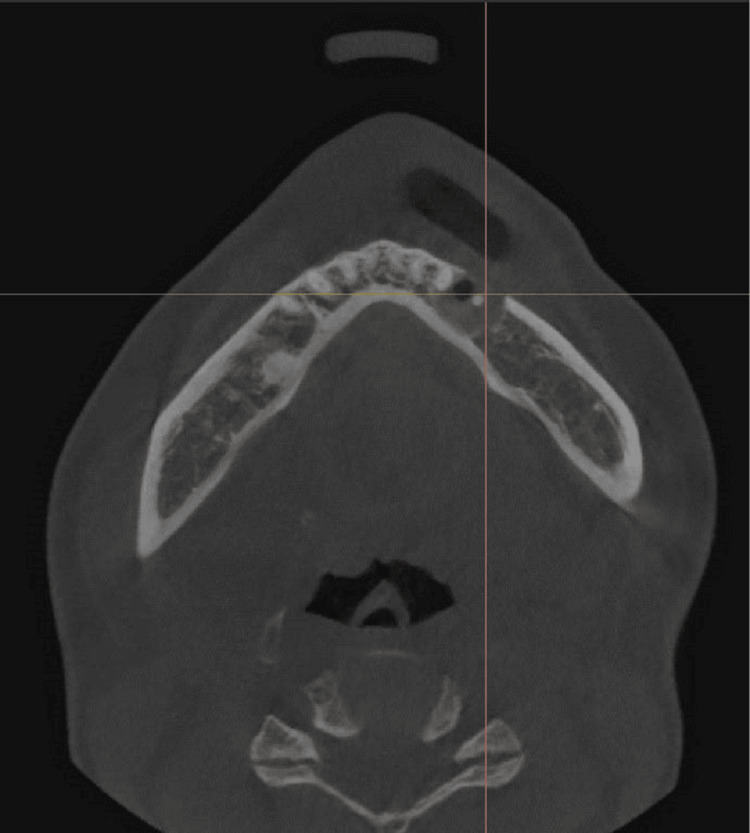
Dental cone beam computed tomography (CBCT) of the lesion A mixed heterogeneous hypodense lesion was noted in the axial view. This hypodense area represents regions where the tissue is less mineralized or where there is a preponderance of fibrous tissue compared to bone. No definite bony expansion was observed, but perforation of the buccal cortex existed.
